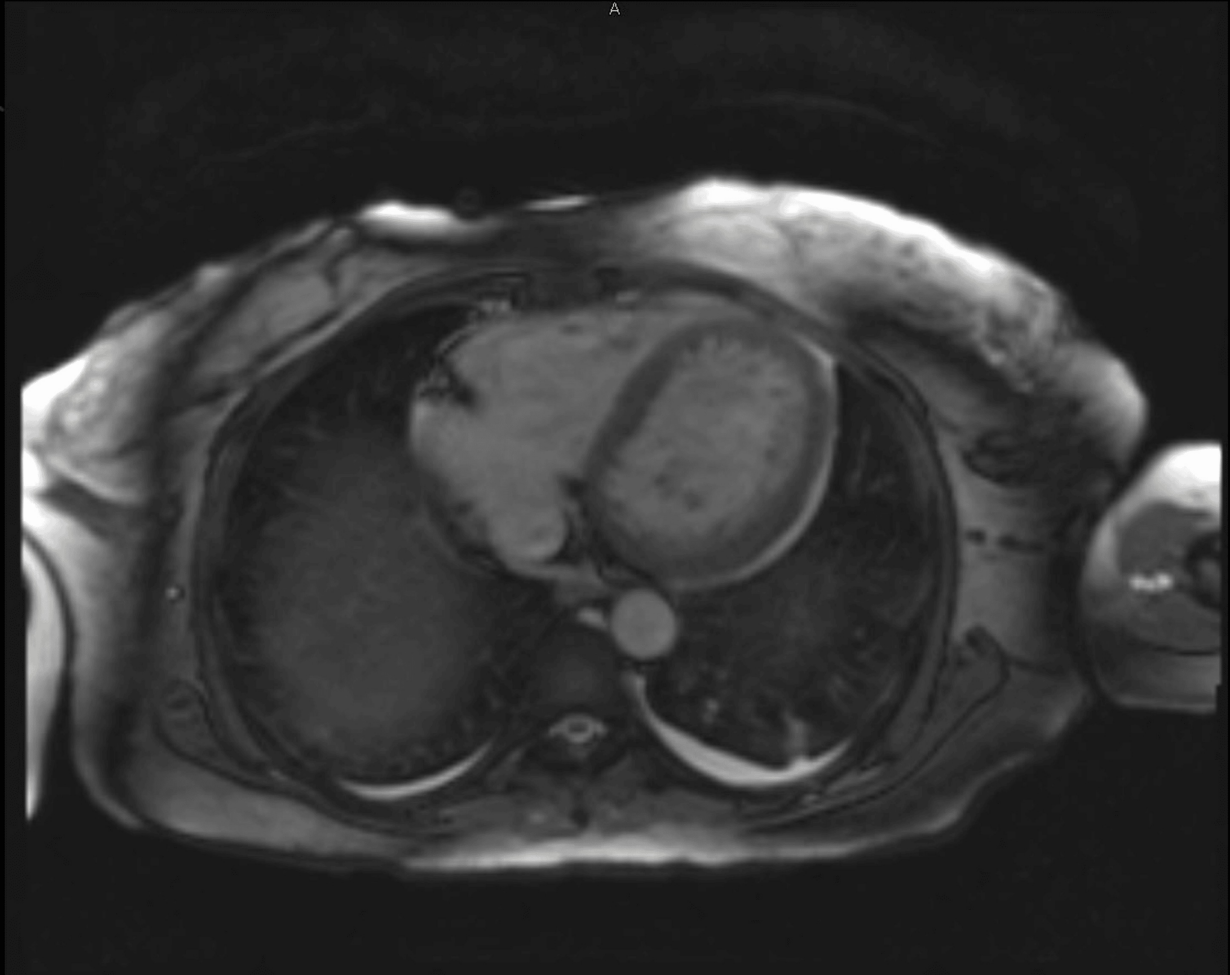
Cardiac MRI ruled out cardiomyopathy MRI: Magnetic resonance imaging
